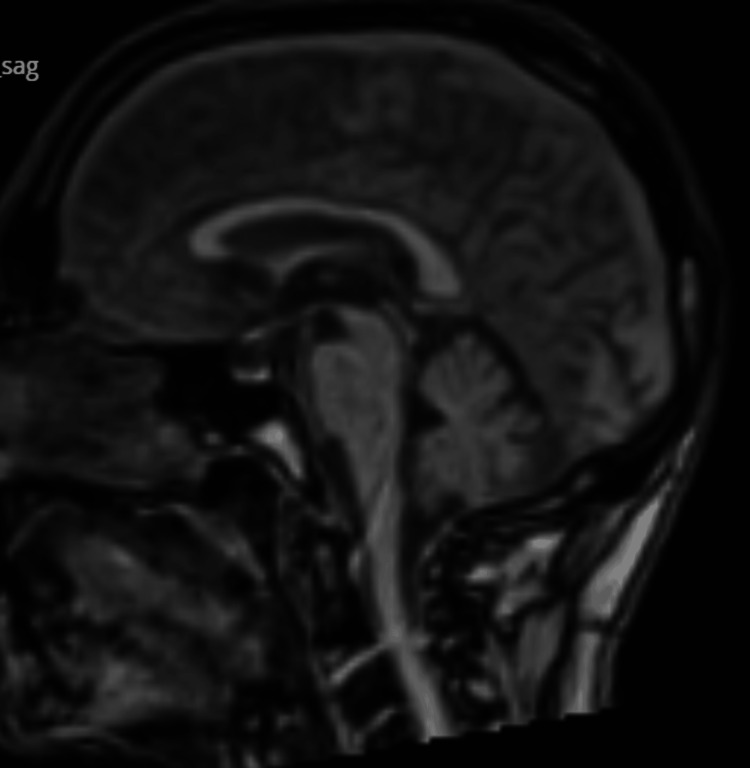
Brain MRI-sagittal section Sagittal T1‑weighted MRI of the brain demonstrates normal anatomy.
